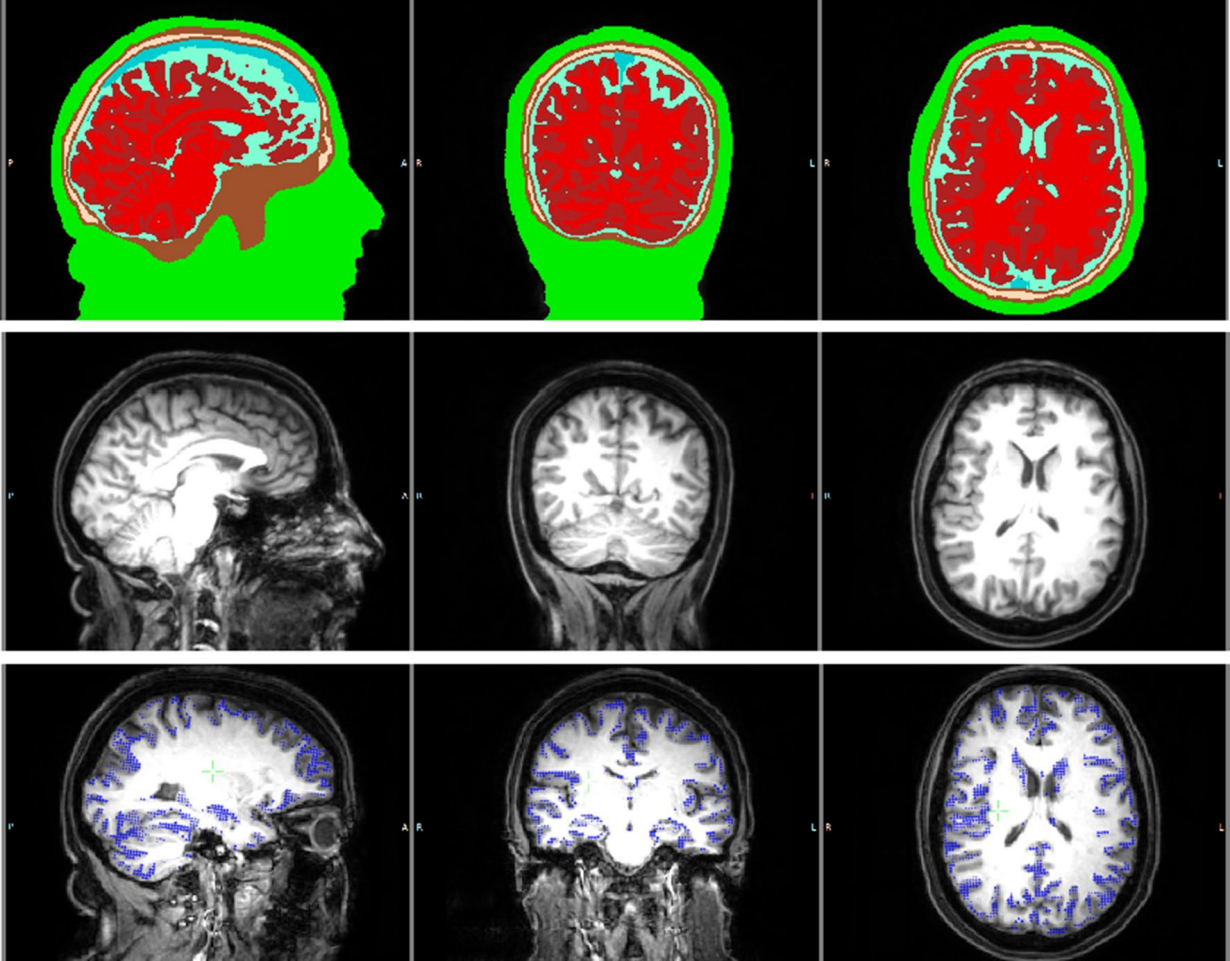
Segmented MRI (*upper row*), T1w MRI (*middle row*) and the source space points (*blue points*) shown on T1w (*lower row*) MRI. Sagittal (*left column*), coronal (*middle column*) and axial (*right column*) slices are shown. Please note that the slices selected in the lower row are different from the top two rows in order to better visualize the source space points. The color codes for the tissues in the segmented MRI are scalp (*green*), skull compacta (*brown*), skull spongiosa (*beige*), dura mater (*dark turquoise*), CSF (*light turquoise*), gray matter (burgundy) and white matter (*red*). *White letters* on the MRIs show the directions (*L* left, *R* right, *A* anterior, *P* posterior). (Color figure online)
